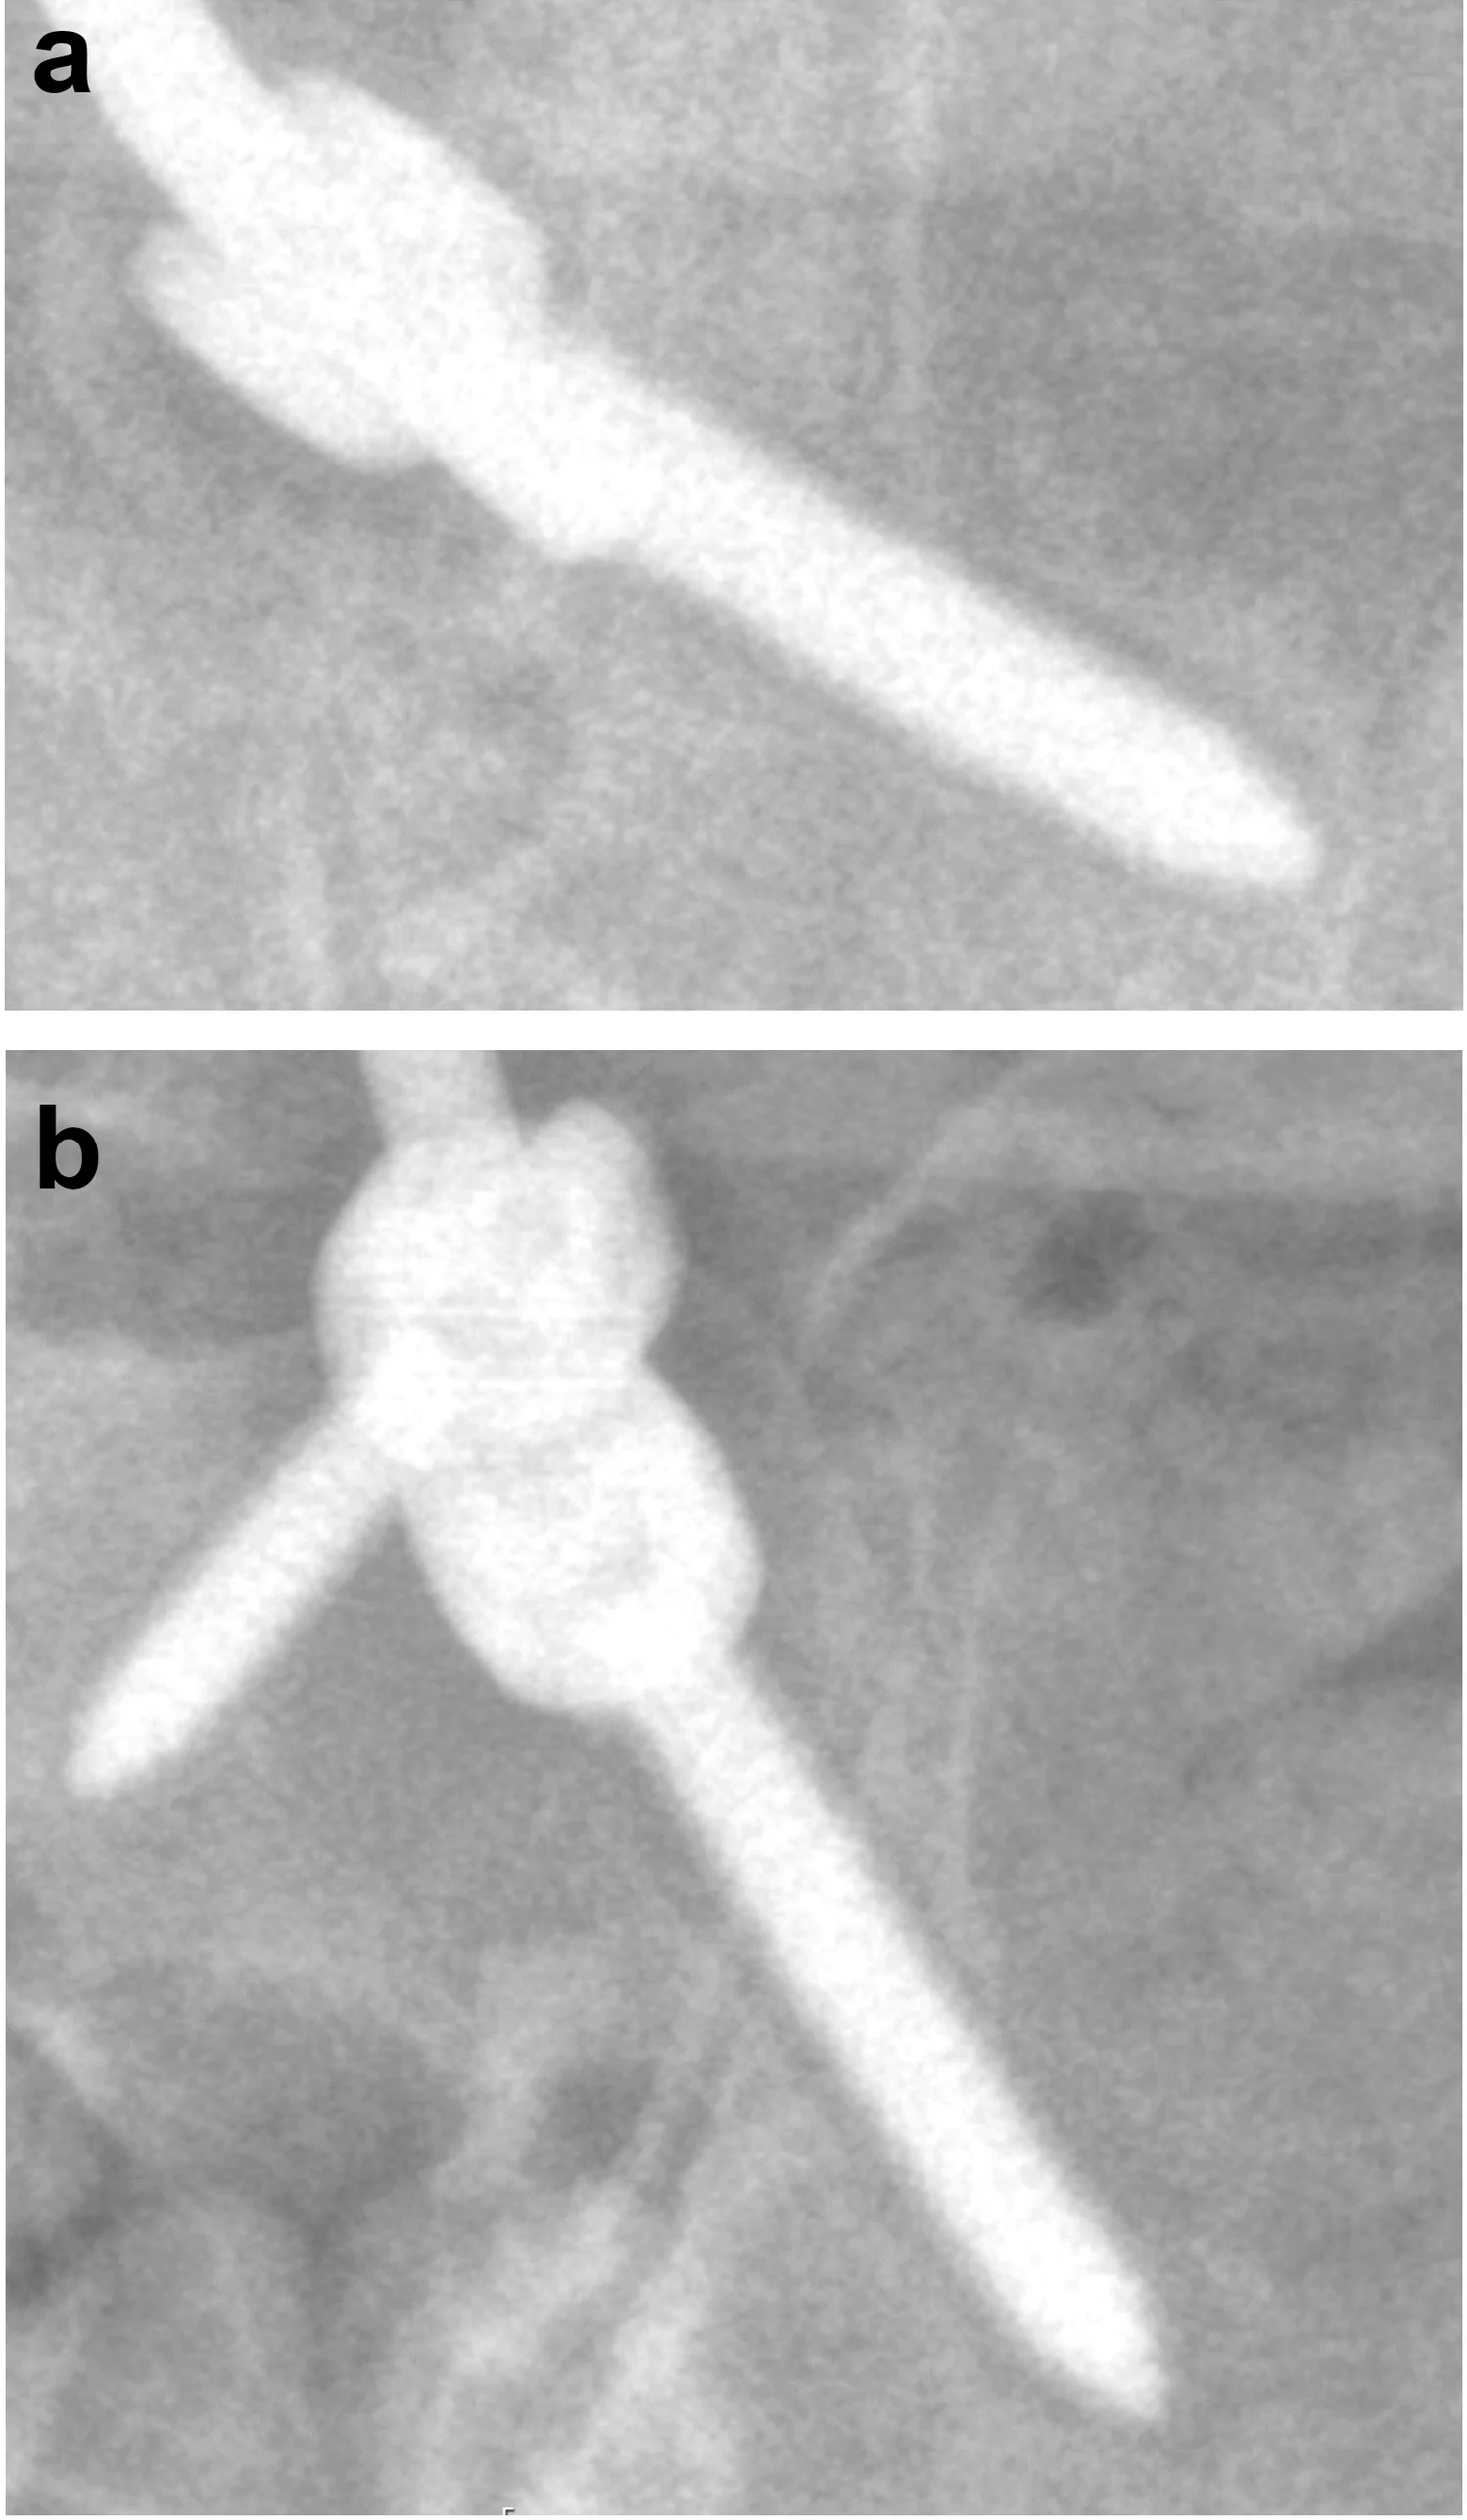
Loosening of spinopelvic fixation. a, b Evidence of pelvic screw loosening with radiolucency surrounding the screw threads on follow-up post-operative standing radiographs
